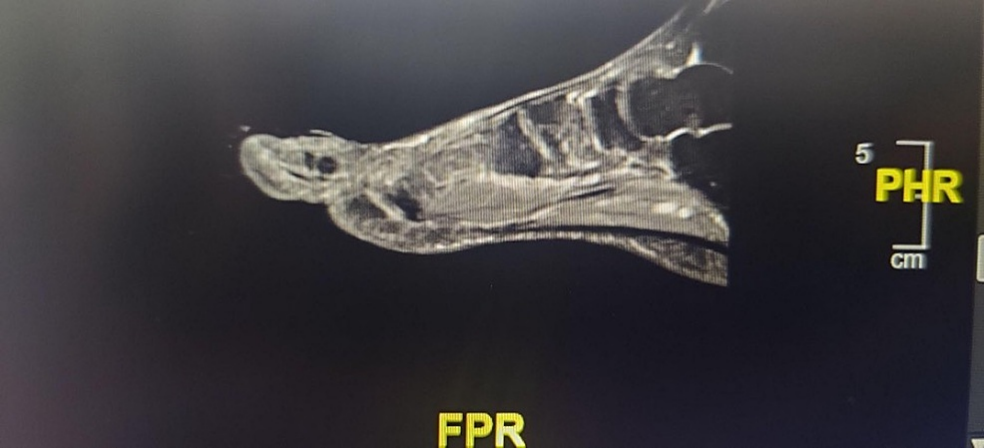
T2-weighted MRI showing soft tissue swelling and ulceration along with near exposure of the bone characteristic for osteomyelitis. MRI: magnetic resonance imaging
